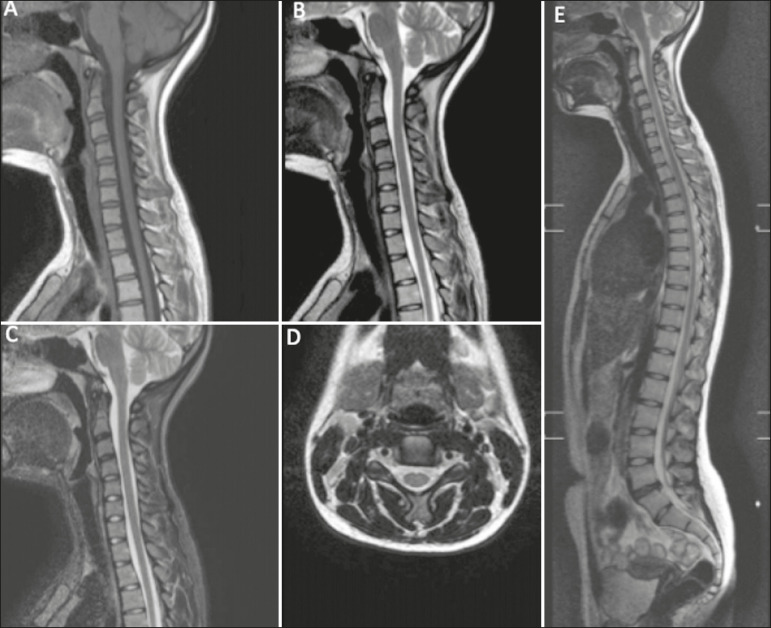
A,B,C: Sagittal T1-weighted, T2-weighted and T2-weighted STIR sequences of the cervical spine, respectively. D: Axial T2-weighted sequence of the cervical spine. E: Whole-spine sagittal T2-weighted sequence, including the base of the skull.
